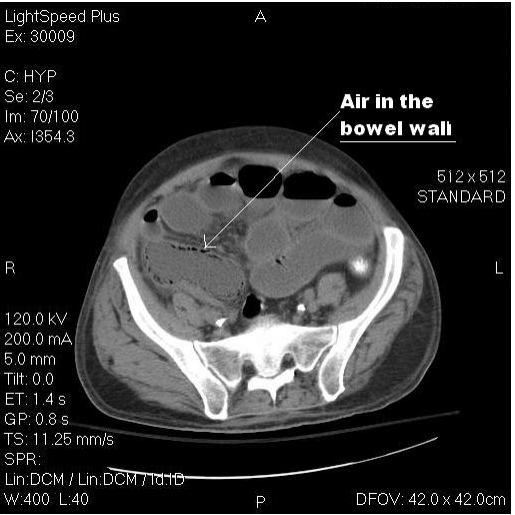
Non contrast computed tomography of the abdomen and pelvis (axial view) shows air in the bowel wall. This radiologic sign is called *Pneumatosis Intestinalis*. There is also evidence of an over-distended ileum, and collapsed left colon suggesting an intestinal obstruction.
